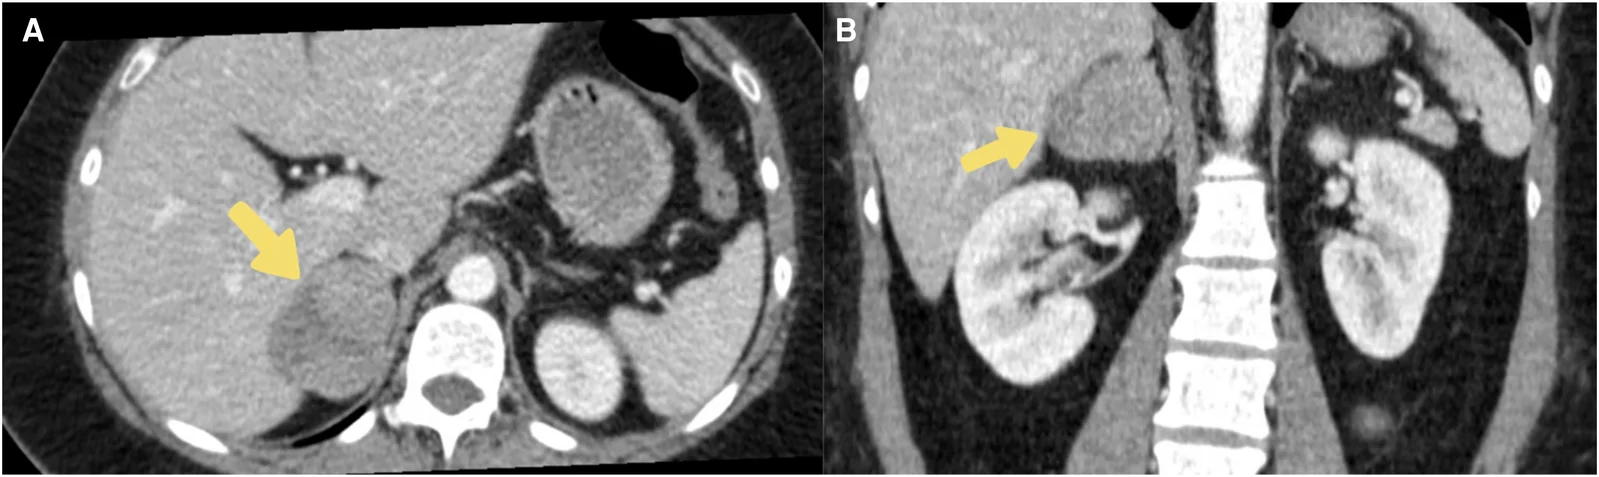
Contrast-enhanced computed tomography (CT) urogram demonstrating a right adrenal mass. A (left, axial view) and B (right, coronal view) show a large, well-circumscribed, heterogeneous mass arising from the medial limb of the right adrenal gland, measuring approximately 5.2 × 4.8 × 4.0 cm, with heterogeneous enhancement (yellow arrows). The mass abuts the inferomedial aspect of the liver. The left adrenal gland appears unremarkable.
